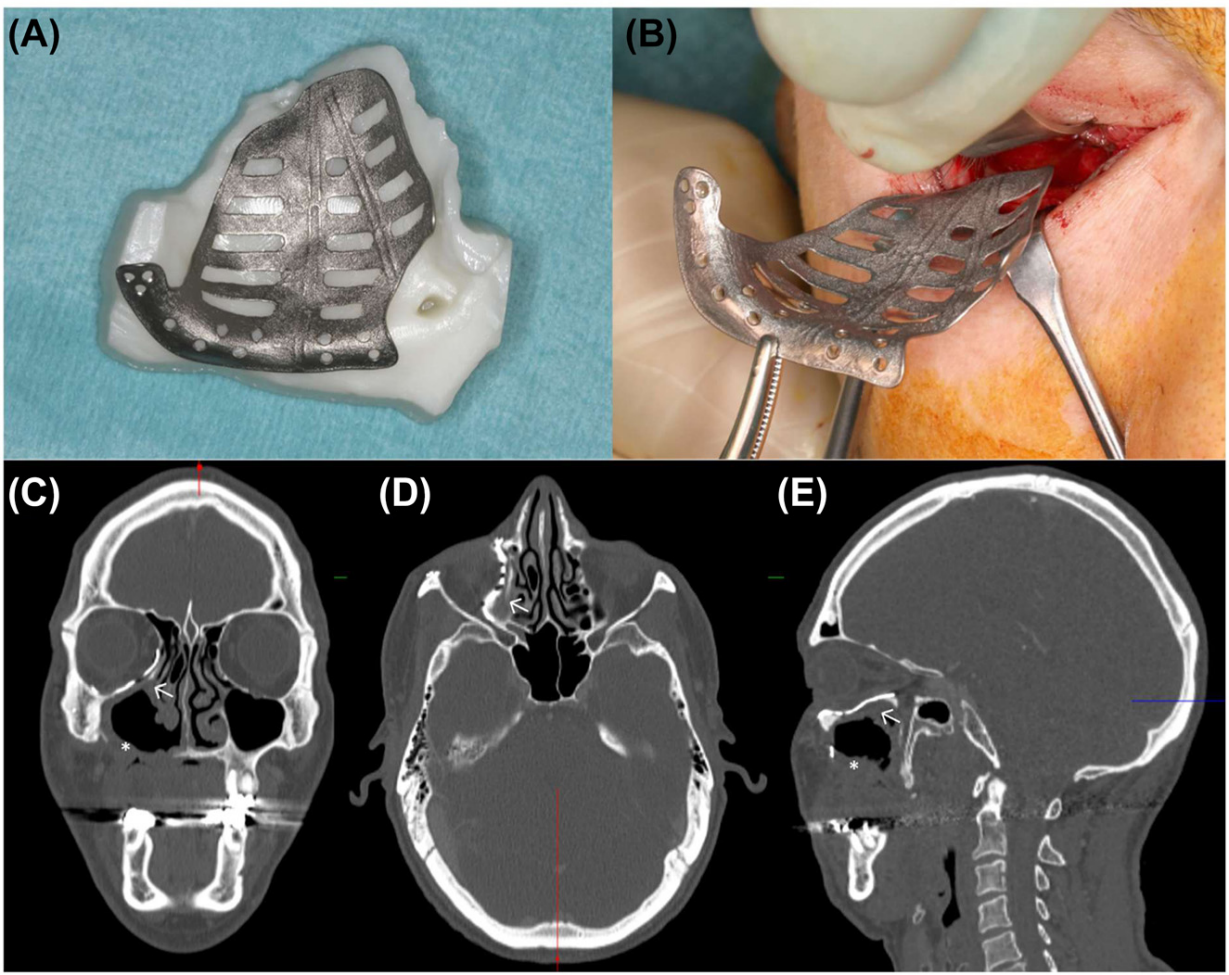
Patient-specific implant (IPS^®^, KLS Martin, Tuttlingen/Germany) on a stereolithography model (A). Implant insertion via an inferior transconjunctival approach (B). CT scan (C–E) before midface reconstruction showing orbital reconstruction with a patient-specific implant (arrows) and midface defect after hemimaxillectomy (asterisk). The complexity of the orbital reconstruction becomes apparent in the various layers: coronal (A), reconstruction of the transition zone, axial (B); reconstruction of the posterior medial bulge and median sagittal (C), reconstruction of the so-called “lazy-s” shape of the orbital floor.
